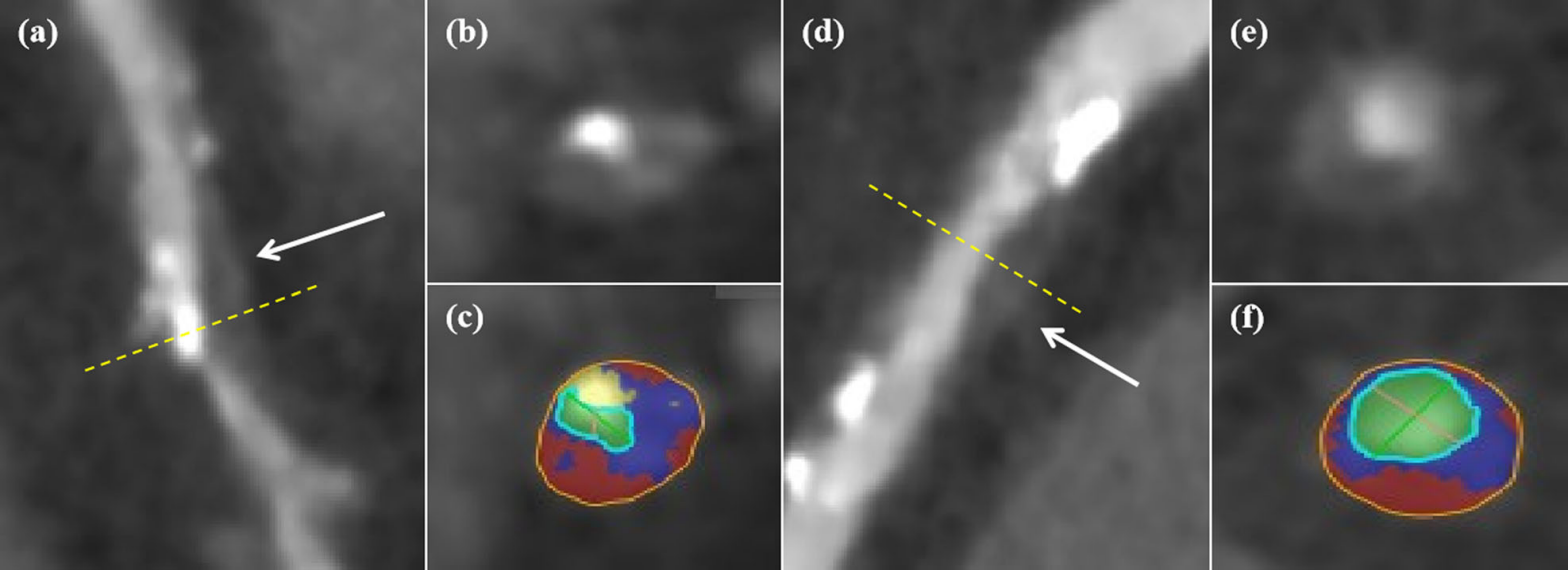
Case examples of CT attenuation values-based coronary plaque classification. Curved multiplanar reformation and axial images of a mixed plaque (a–c) and a non-calcified plaque (d–f) are shown. Lumen area (green), necrotic content (red), fibrous content (blue) and calcified content (yellow)
